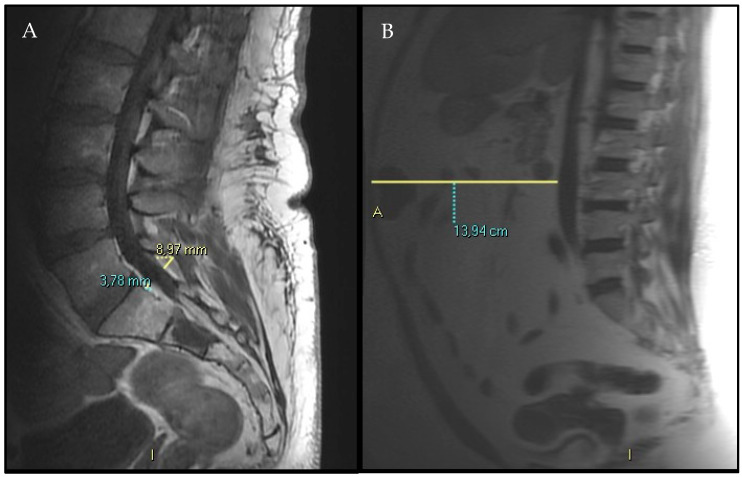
Exemplification of epidural fat (EF) and visceral adipose tissue (VAT) measurement on MRI studies (A). T1-weighted sagittal sequences were used to assess anterior and posterior EF thickness within the SC, measured in a plane parallel and tangent to the superior endplate of the S1 vertebral body. (B) MR-localized sequences were employed to evaluate VAT deposition, which is the distance between the abdominal muscular fascia and the anterior wall of the abdominal aorta at the L3 vertebral level. Please note that commas (,) have been used as decimal signs in the figures measurements.
